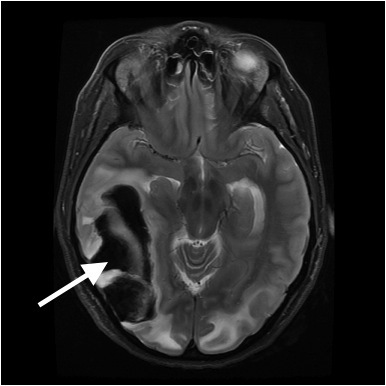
T2 weighted magnetic resonance imaging of Patient 3 on hospital day 4 with massive right-sided temporo-occipital intraparenchymal hemorrhage.
